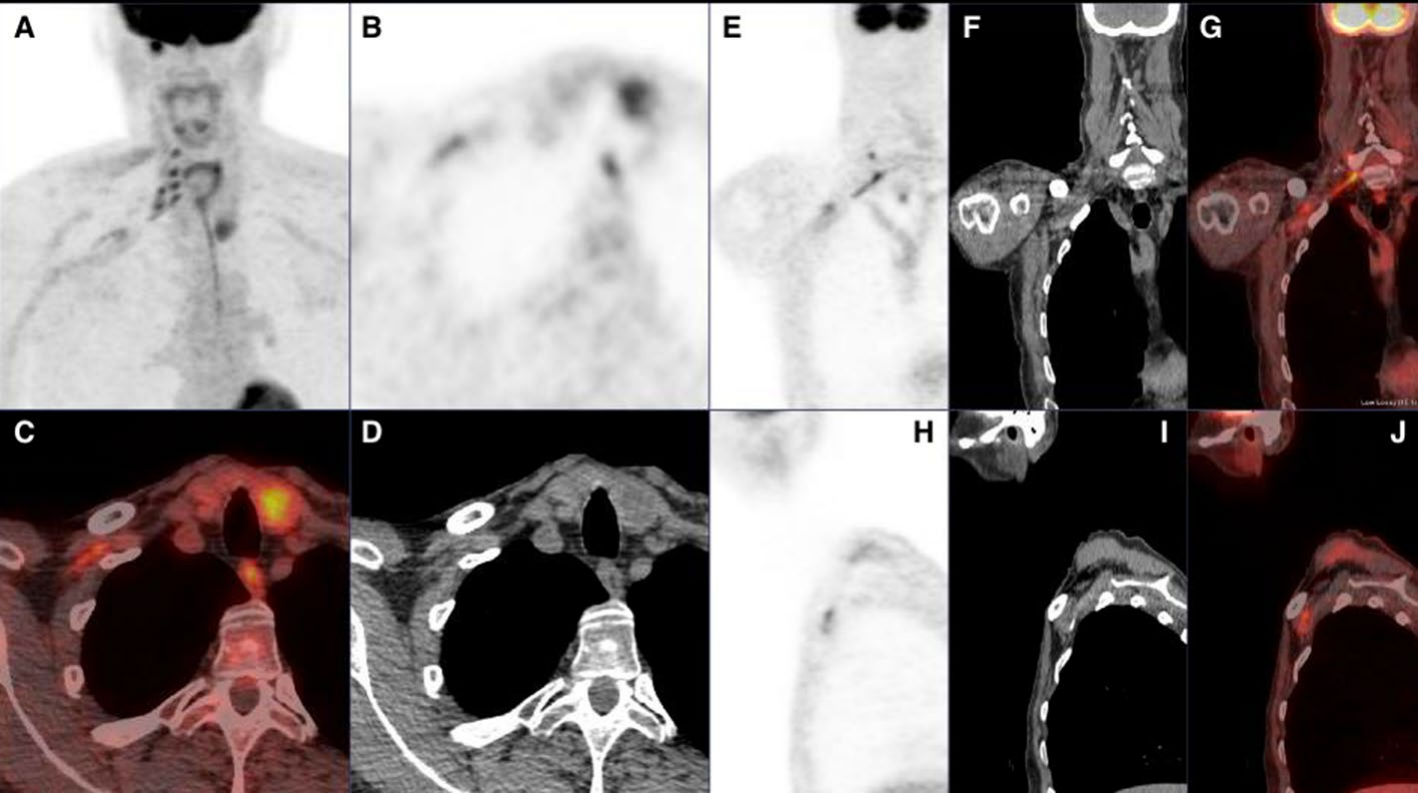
Axial PET, fused and CT images (B, C and D) at the level of the right clavicle. Note intense uptake by the trunks and cords. Coronal PET, CT and fused images (E, F and G) and Sagittal PET, CT and fused images (H, I and J) confirm the uptake in the distribution of the brachial plexus
